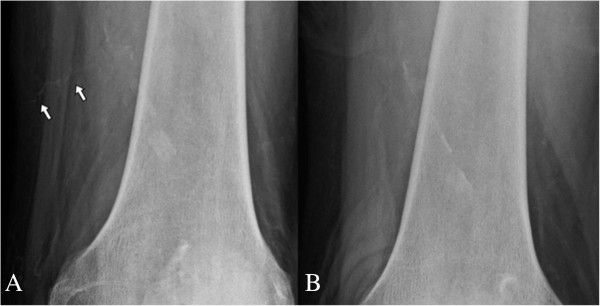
Plain radiographs of the right thigh. (A) A calcified subcutaneous vessel (arrows) presented before specific treatment. (B) The calcified small vessel was completely absent after 11 months.
